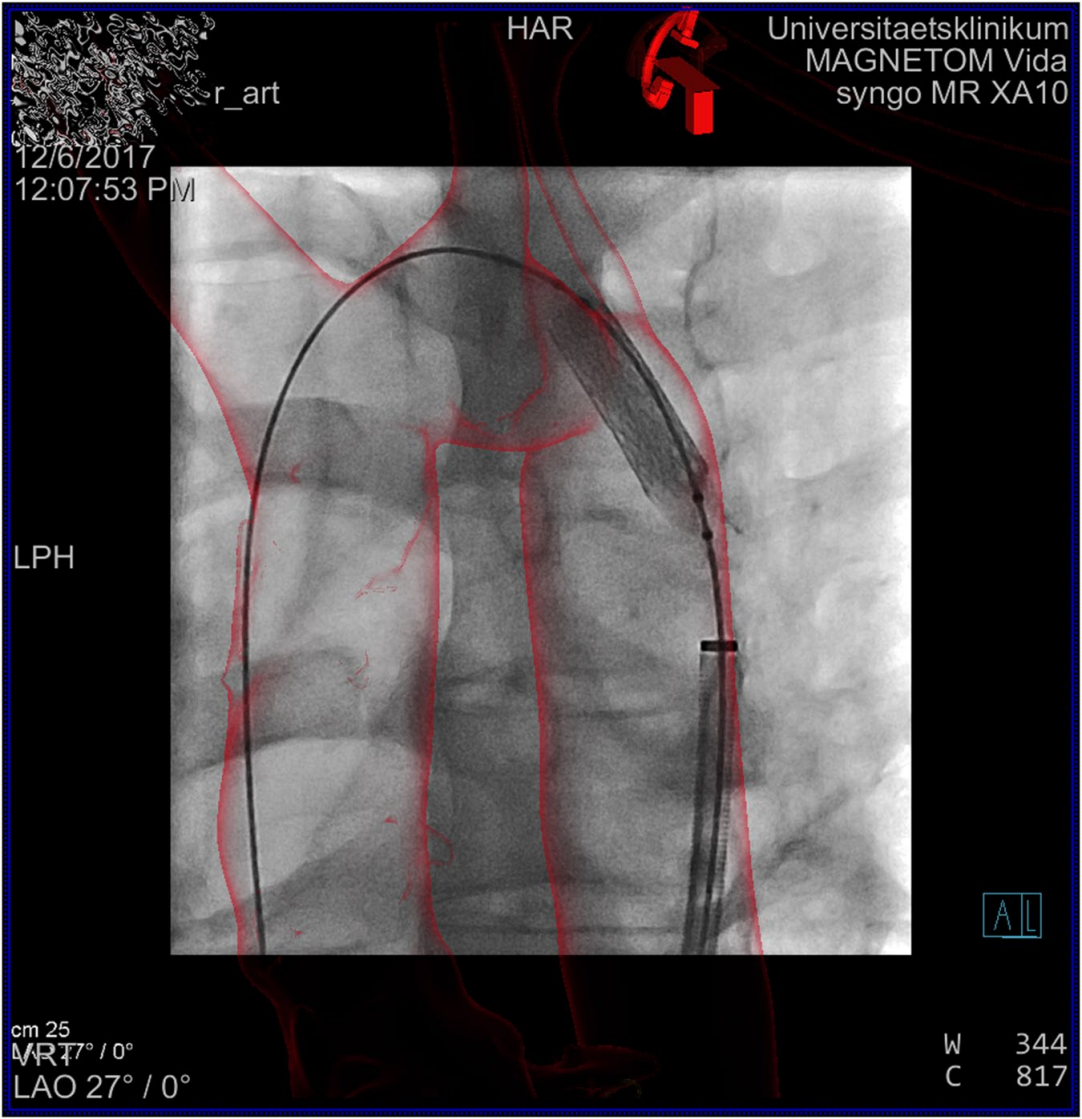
Adult patient with coarctation of the aorta; overlay of a 3D angiography produced by MRI. It is reconstructed in stereolithographic format. 3D-guidance is used for stenting the coarctation.
